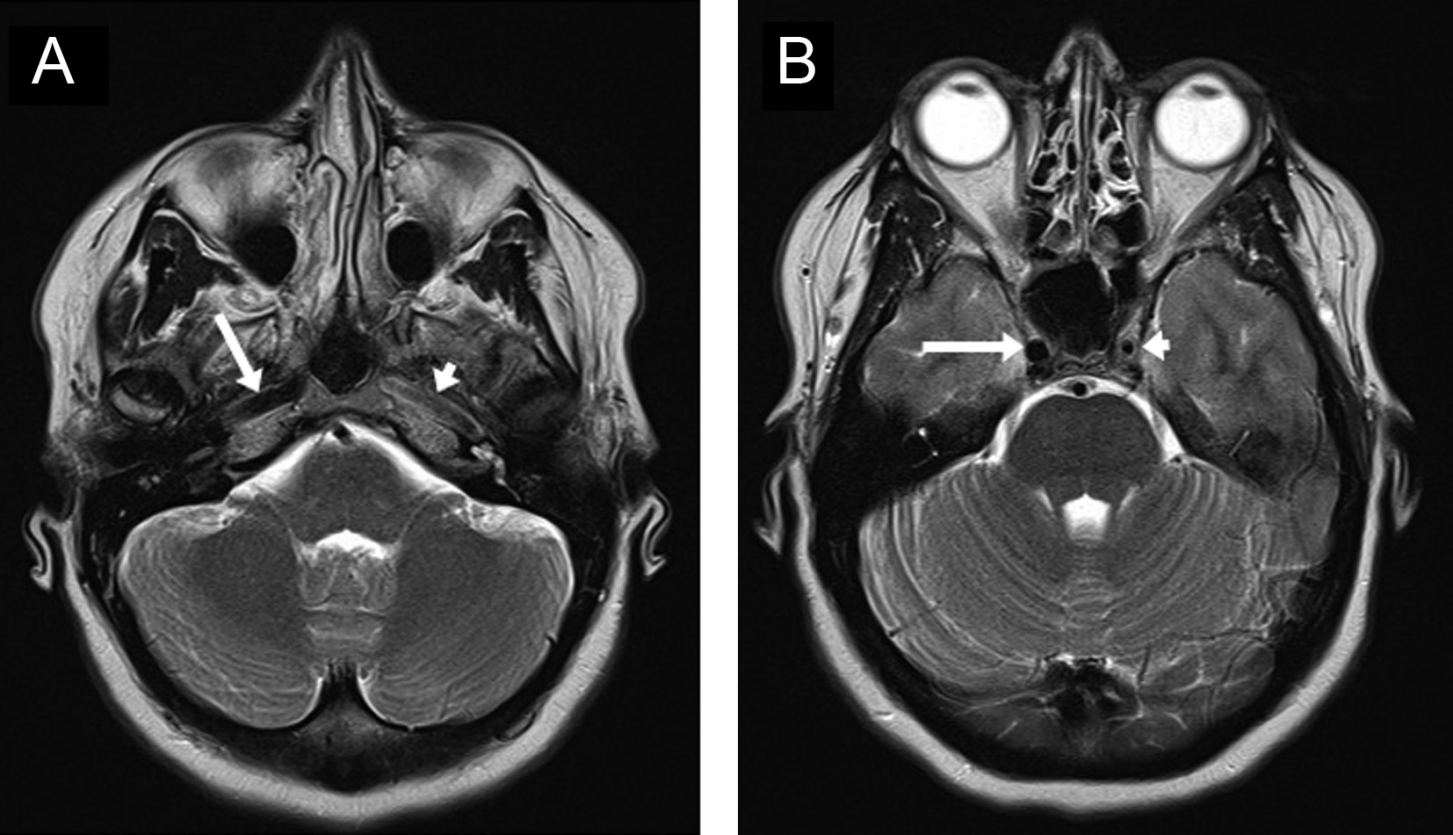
Axial T2-weighted MRI demonstrating dissection of internal carotid artery. (A) The petrous left internal carotid artery (short arrow) has diminished flow, demonstrated by a loss of black signal, compared to the normal right internal carotid artery (long arrow). (B) The cavernous left internal carotid artery (short arrow) with a smaller lumen compared to the normal right internal carotid artery (long arrow).
